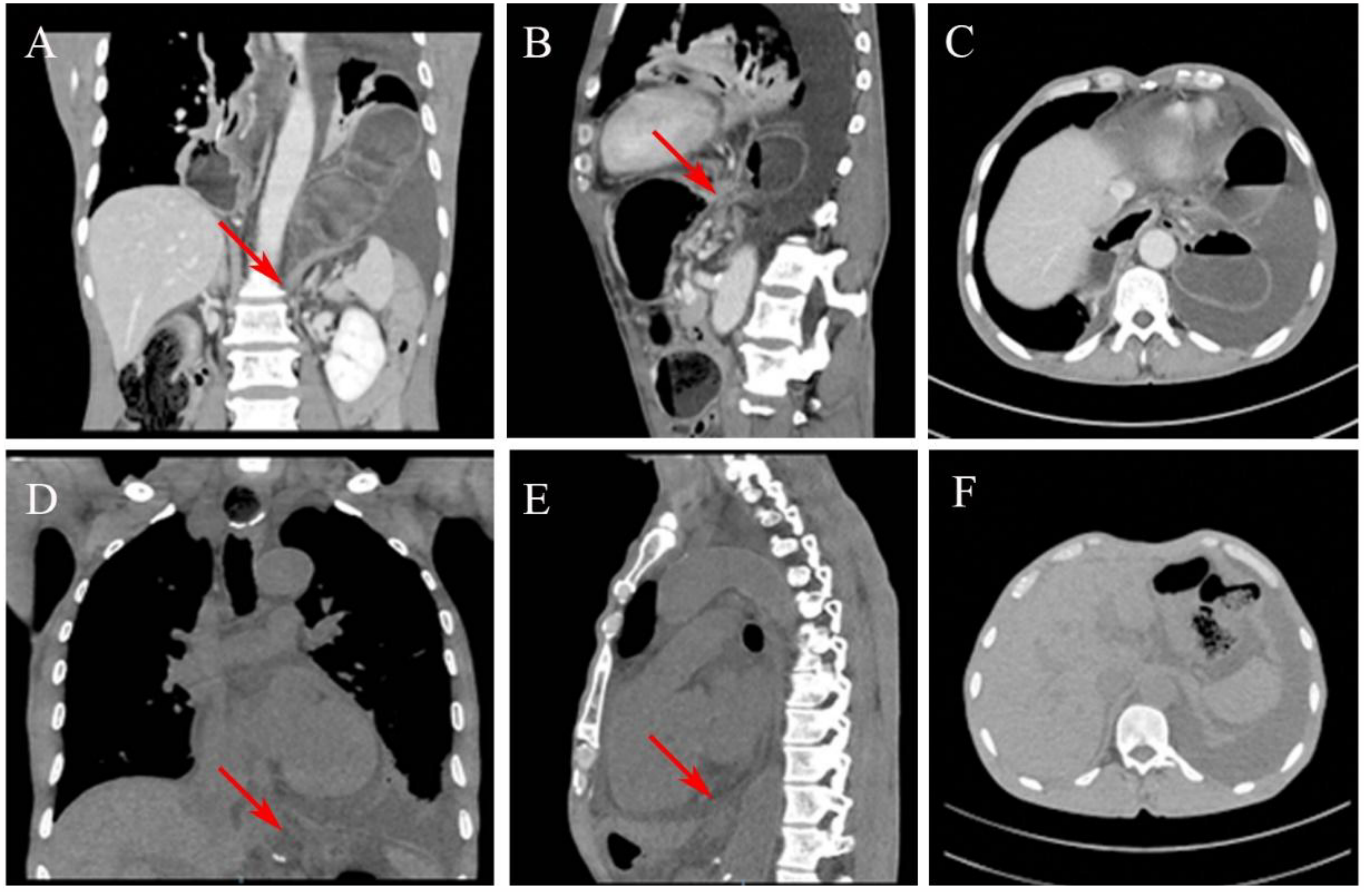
CT images showing the transverse colon herniating through the esophageal hiatus. (A) Coronal view and (B) sagittal view reveal the herniation into the thoracic cavity. (C) The hernia is less distinct on the axial view. (D,E) A review of the coronal and sagittal images from the initial chest CT shows a small amount of abdominal fat herniating through the esophageal hiatus into the thoracic cavity. No herniation is visible on the axial view (F).
